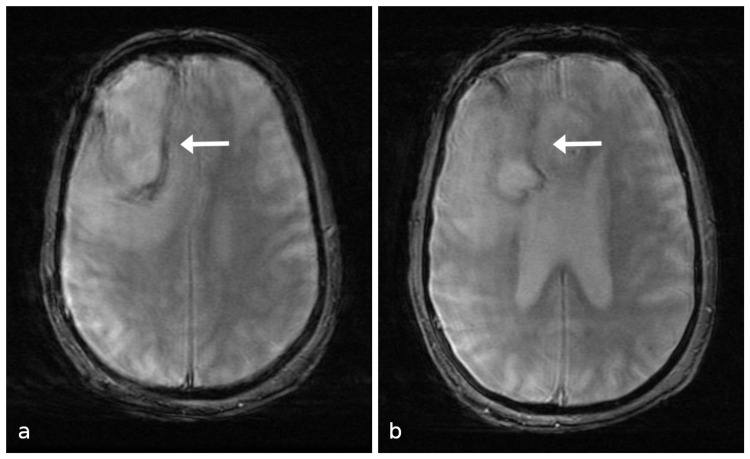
(a) and (b) MRI GRE sequences showing some susceptibility artifact in the periphery of the lesion with little to none within the lesion. MRI, magnetic resonance imaging; GRE, gradient echo
